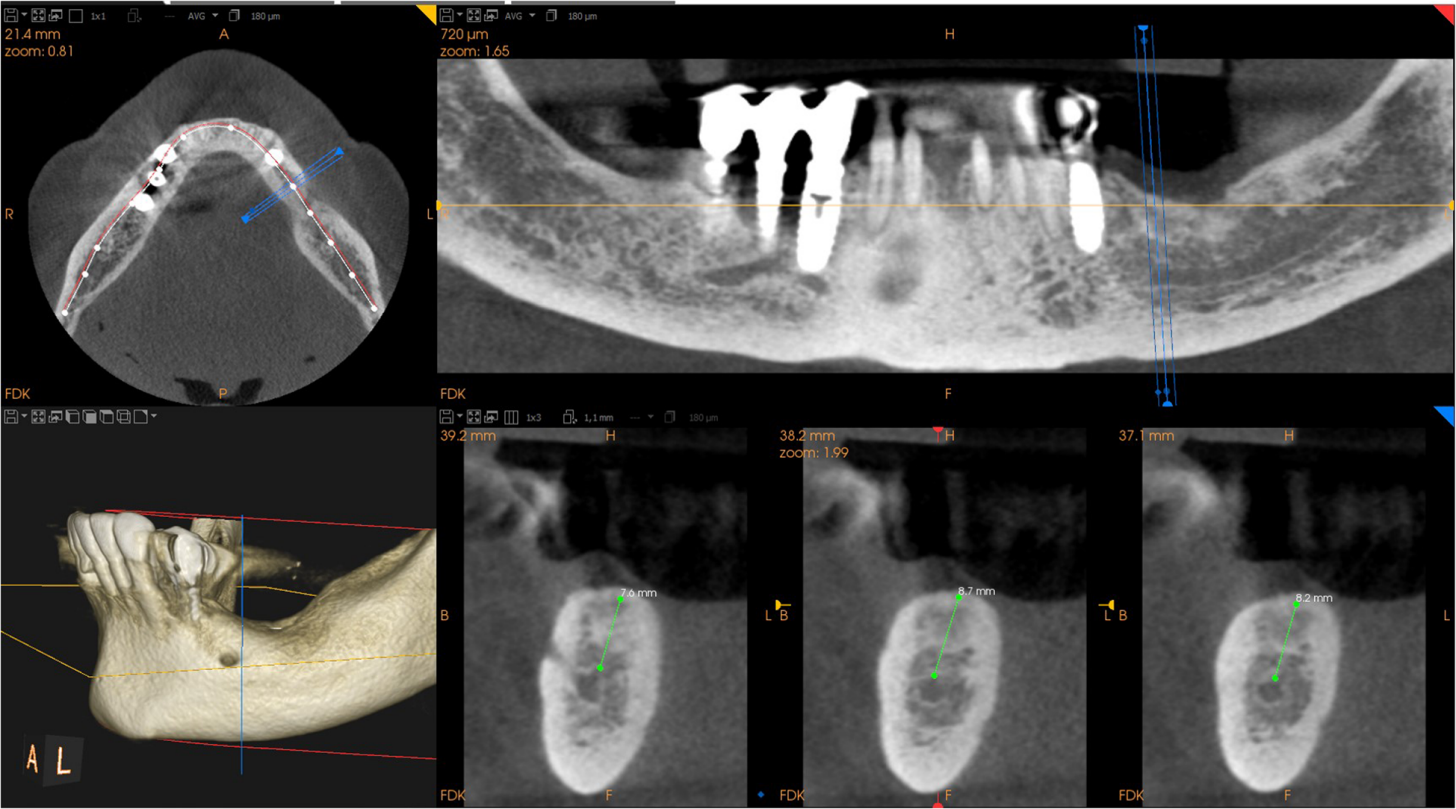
Initial CBCT (CS 9300®, Carestream Dental, Atlanta, GA, USA) that revealed a limited bone height (< 8 mm from the top of the crest to the inferior alveolar nerve) in the posterior left mandible. The 69 years old female patient was referred to our clinic and since she had experienced in the past failures with bone regenerative therapies, she did not want to undergo bone regeneration; the placement of a standard length endosseous implant was therefore not possible, and the only possibility was the placement of an extrashort (6 mm) endosseous implant
